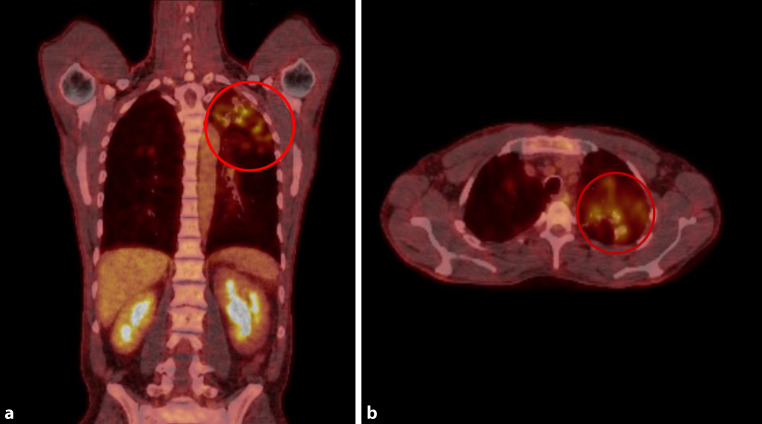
PET CT scan images of coronal (a) and axial (b) planes showing high metabolic activity in the pulmonary lesions in the left upper lung lobe (*red circles*) with a maximum standardized uptake value (SUVmax) of 4.0–6.4. The high metabolic activity in the abdomen is a normal finding and represents the kidneys
